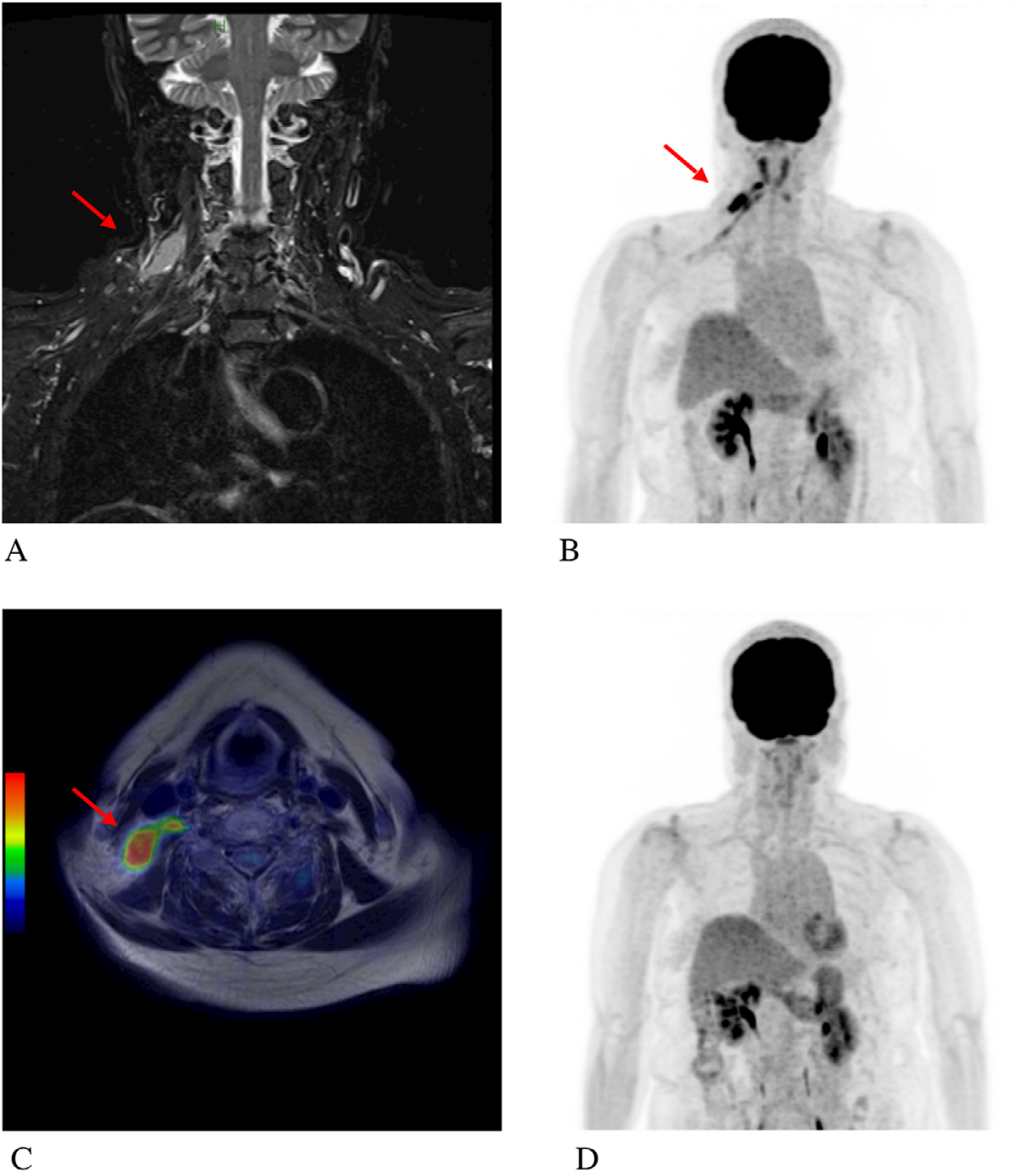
A 75‐year‐old woman was diagnosed with primary breast diffuse large B‐cell lymphoma, non‐germinal centre B‐cell‐like type (stage IE). She was treated using three cycles of rituximab, cyclophosphamide, doxorubicin, vincristine, and prednisolone, followed by radiation therapy (30 Gy/10 fractions). Thereafter, complete metabolic response (CMR) was confirmed by ^18^F‐fluorodeoxyglucose (FDG)‐positron emission tomography (PET)/computed tomography (FDG‐PET/CT). Three months after CMR was confirmed, she developed pain in her right shoulder. Although FDG‐PET/CT was performed, no abnormal findings were noted and she was followed up in an outpatient setting. Six months later, she had difficulty lifting her right upper limb due to pain in her right shoulder. A manual muscle test revealed 2/5 (deltoid), 4/5 (biceps), and weakness of the right upper limb. Coronal short TI inversion recovery magnetic resonance imaging (MRI) demonstrated thickening of the right brachial plexus (Panel A). In addition, abnormal uptake of FDG (SUVmax 12.21) in the right brachial plexus was noted on FDG‐PET/MRI (Panels B and C). No abnormal accumulation of FDG was found in the right breast as a primary involved site and cerebrospinal fluid tests were negative for lymphoma cells. A diagnosis of neurolymphomatosis was made based on her medical history, and MRI and FDG‐PET/MRI findings. Three cycles of high‐dose methotrexate therapy (3.5 g/m^2^) plus rituximab were performed, and CMR was achieved (Panel D).
